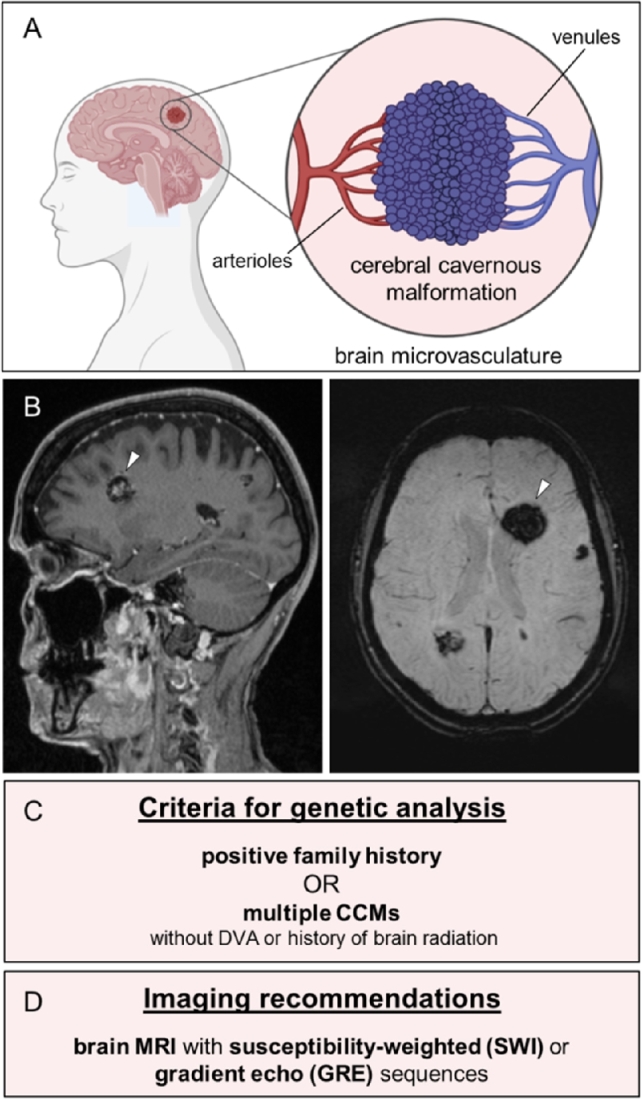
Clinical features of CCMs. (A) CCMs are mulberry-like vascular lesions in the microvascular bed of the central nervous system. (B) Sagittal T1-weighted (left) and axial susceptibility-weighted magnetic resonance images (right) show a large CCM (white arrowhead) and multiple smaller CCMs in both hemispheres of a patient with a pathogenic *CCM1* germline variant. (C) Criteria for genetic testing of patients with CCMs (adapted from [2]). DVA = developmental venous anomaly. (D) Recommended imaging techniques for diagnosis or follow-up of CCMs (adapted from [2]).
